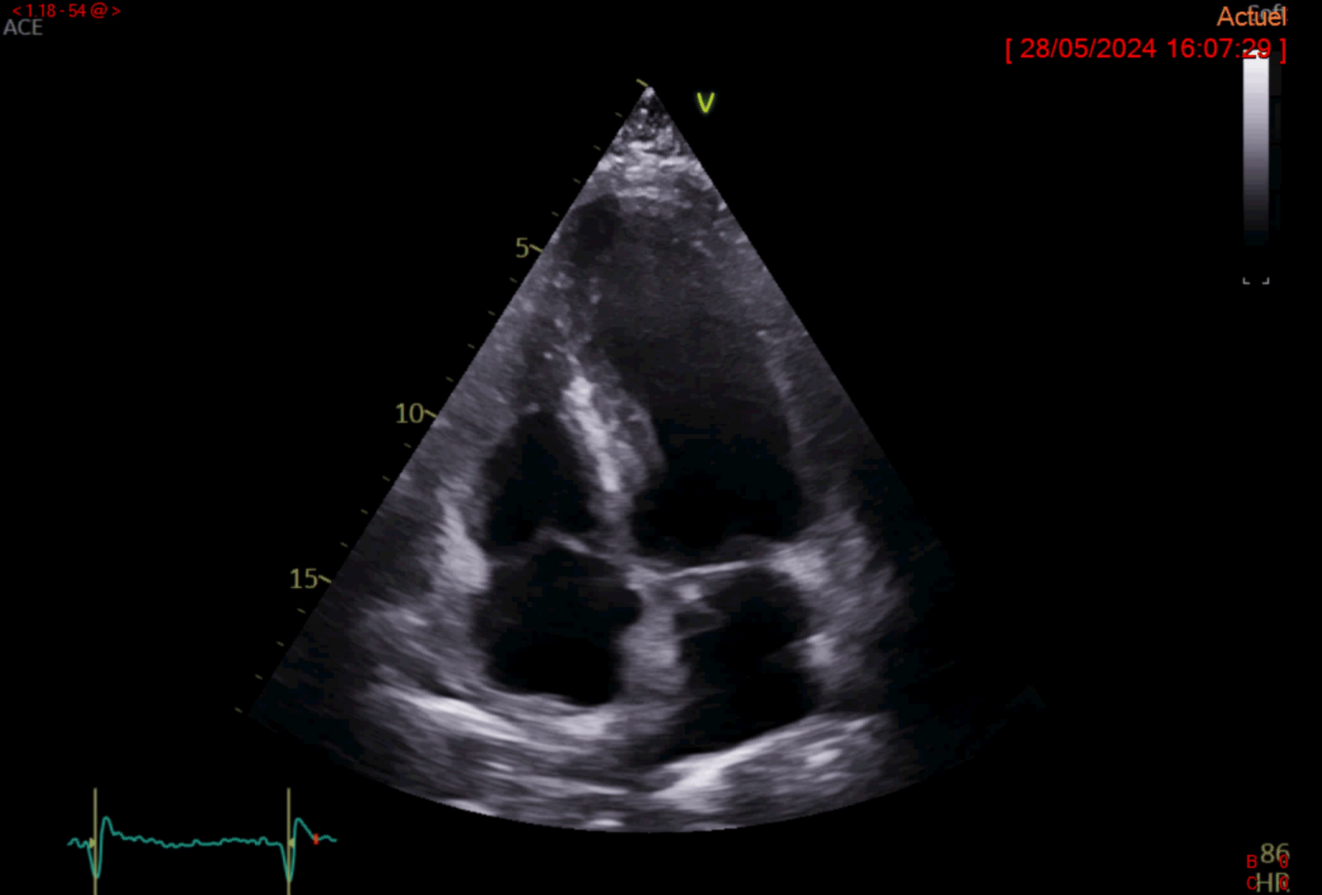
Transthoracic echocardiography Transthoracic echocardiography revealed concentric left ventricular hypertrophy with a septal diastolic thickness of 11.5 mm and a septal bulge thickness of 17 mm. The left ventricular ejection fraction (65%) was preserved.
